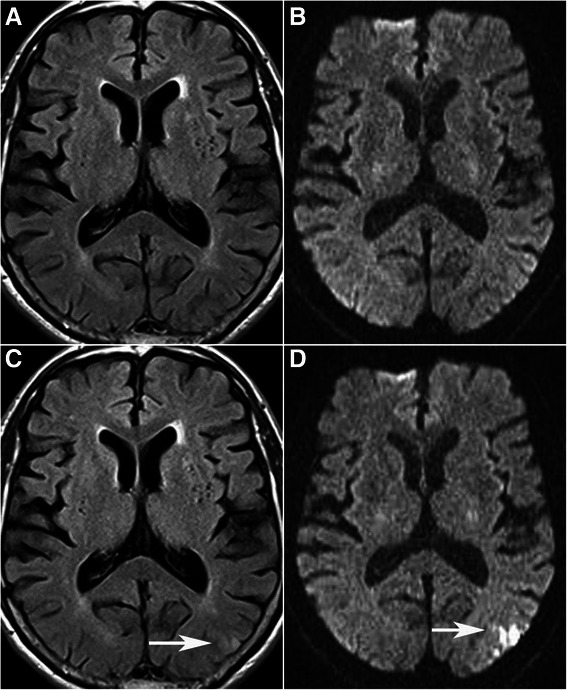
Seventy-four-year-old man with 70 % stenosis of left ICA. Preoperative (a) FLAIR and DWI (b) images show no abnormality. Postoperative FLAIR (c) and DWI (d) images demonstrate new left parietal small acute ischemic lesion (*white arrow*) which is not seen on preoperative images (a, b)
